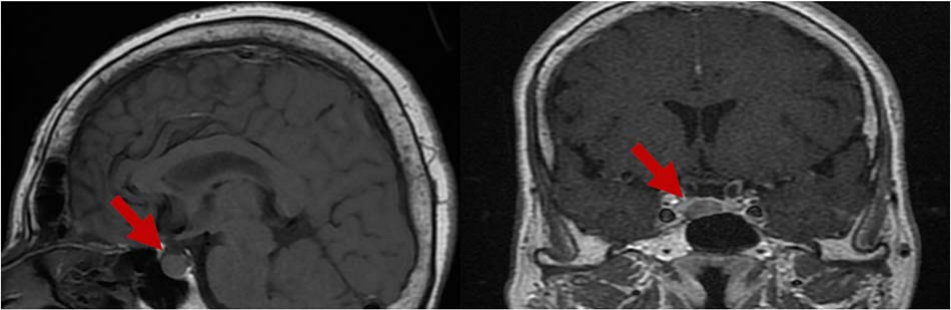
Pituitary MRI. Identification of of a somatotroph adenoma (red arrows).
